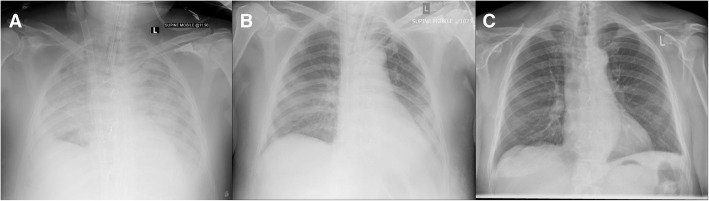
Chest X-Rays performed: (a) after VV-ECMO insertion: patchy airspace of opacification bilaterally. Features in keeping with ARDS; (b) 6 days after VV-ECMO insertion, immediately before weaning: significant improvement in the bilateral perihilar pulmonary infiltrates; (c) at three-months follow up: heart size normal, lungs and pleural spaces clear
